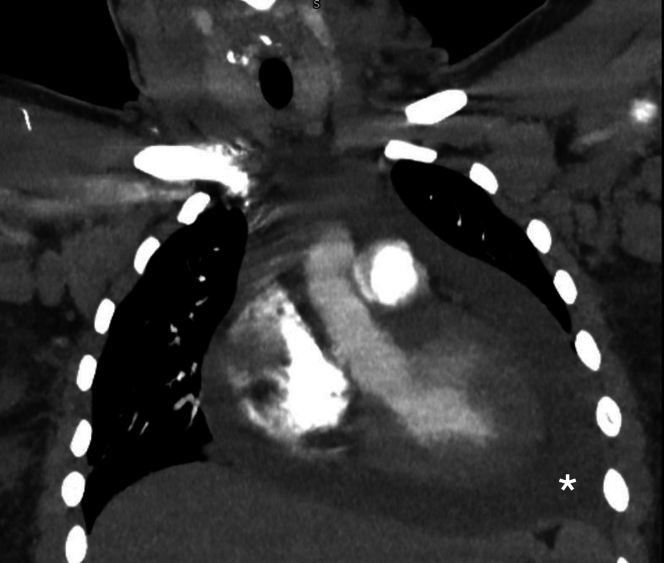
CT Pulmonary Angiogram The CT pulmonary angiogram excluded pulmonary embolism but did confirm a 3-cm pericardial effusion (asterisks), pulmonary edema, contrast reflux into the inferior vena cava, and diffuse lymphadenopathy. CT = computed tomography.
